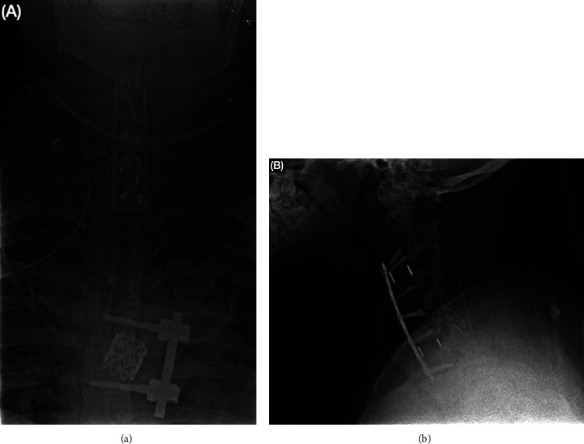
Postoperative x-ray of the cervical spine: (a) surgical material consisting of a 64 mm titanium plate and transcortical locked screws placed from C3 to C7; (b) intersomatic cages at C3-C4 and C6-C7 levels. The amplitude of the intervertebral spaces C2-C3, C4-C5, and C5-C6 is reduced.
